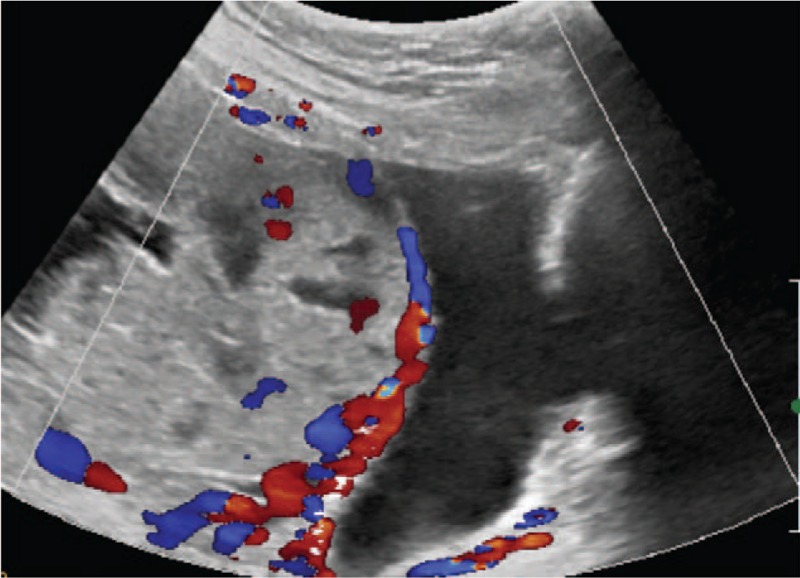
Ultrasound images before cesarean section. It showed placenta, fluid dark areas in placenta, and rich blood flow between placenta and anterior wall of lower segment of uterine.
